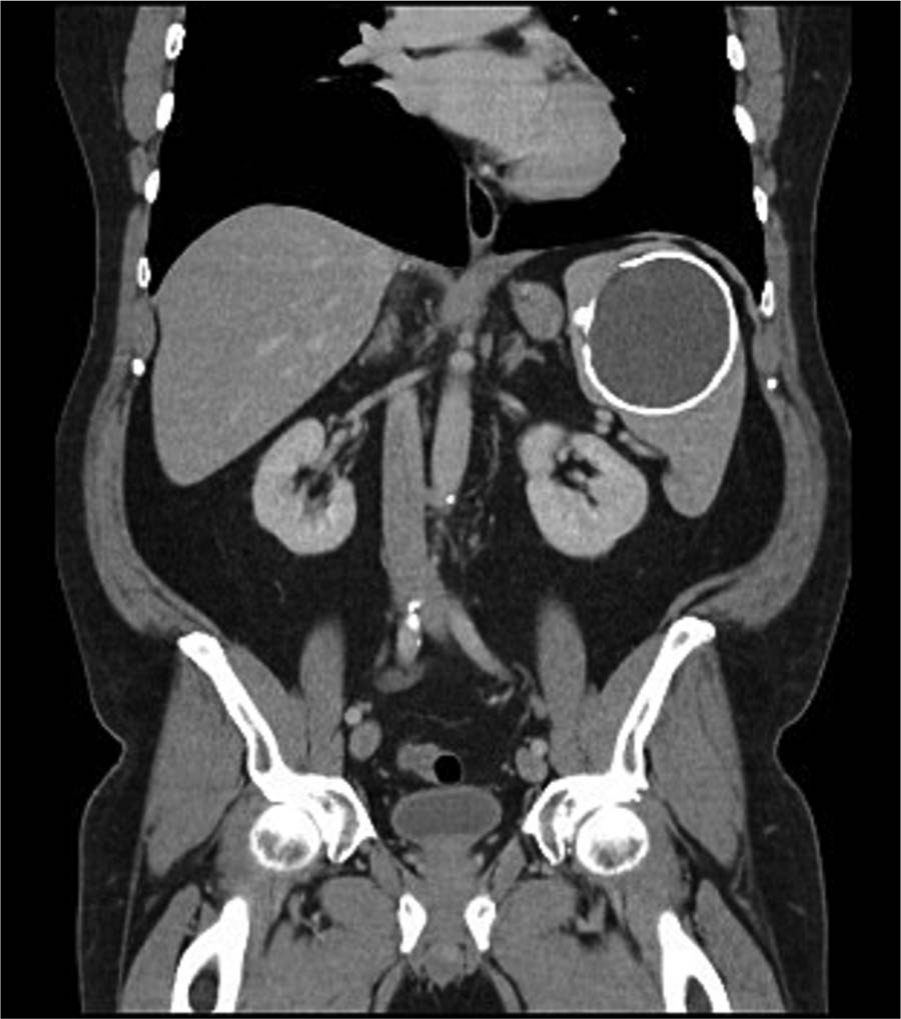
Coronal portal venous phase CT scan of the abdomen and pelvis demonstrating the calcified splenic lesion.
